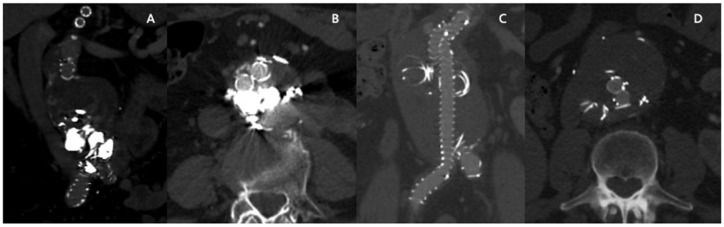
(A,B) Artifacts in CTA after EVAR with sac embolization by platinum coils. (C,D) CTA shows clear images and sac high definition after embo FEVAR with MReye deployment.
